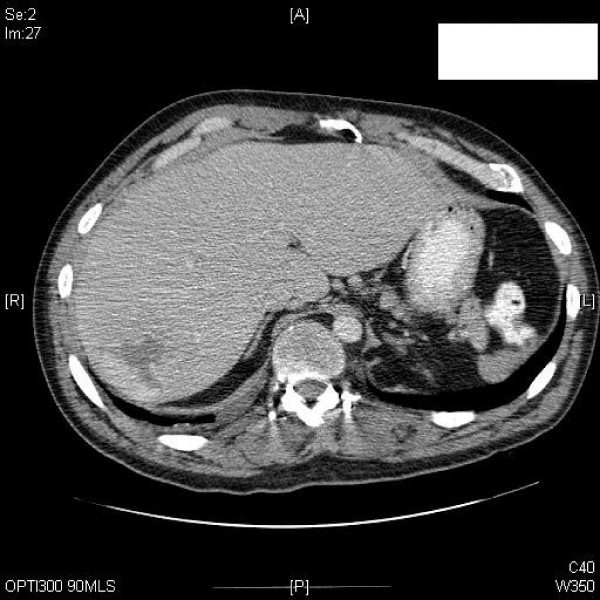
Axial CT – portal venous phase shows increased enhancement of the haemangioma.
